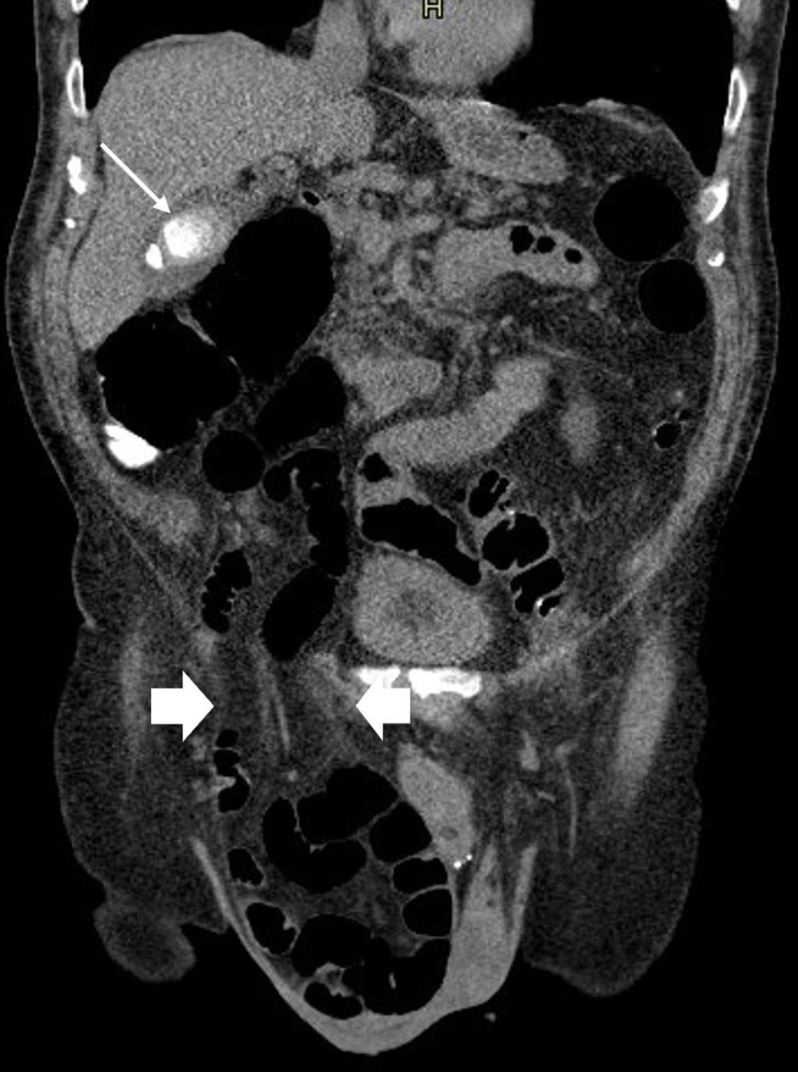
Coronal section of the previous unenhanced CT colonoscopy which demonstrated large and small calcified gallstones in the gallbladder (thin arrow) and a large right inguinoscrotal hernia containing small bowel loops (arrow heads delineating the neck).
